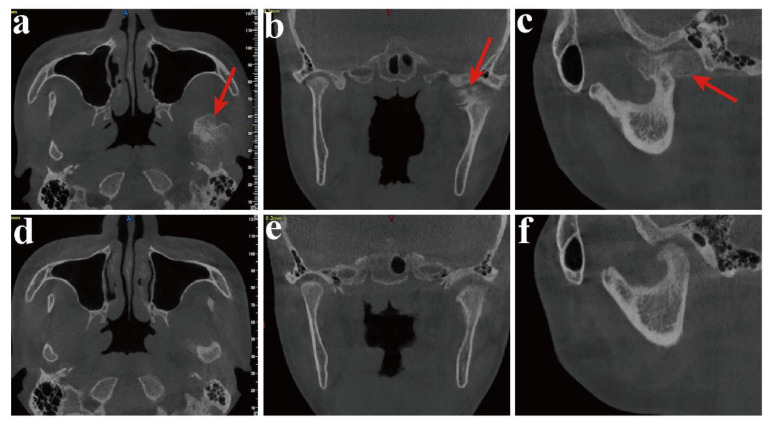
The change in the 2D CT image preoperatively and 1 year after surgery: (a,d) axial CT scan,, preoperatively and postoperatively; (b,e) coronal CT scan, preoperatively and postoperatively; (c,f) sagittal view, preoperatively and postoperatively (red arrow—condylar OC).
